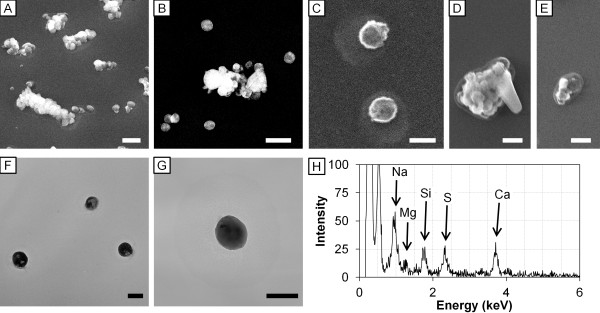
FE-SEM images, TEM images and EDS spectrum of particles released from ultrasonic humidifier. FE-SEM images of particles (0.5 − 2.5 μm) released from the humidifier with (A) tap water and (B) high-silica water under humidity 75%; particles (<0.5 μm) from the humidifier with tap water under (C) humidity 75% and (D, E) 99% are shown. TEM image of particles (<0.5 μm) from the humidifier with (F) tap water and (G) high-silica water under humidity 75%. Scale bars represent (A, B) 1 μm, (C, F, G) 200 nm, and (D, E) 500 nm. (H) EDS spectrum of the particle (C) is shown. The peaks of Na, Mg, Si, S, and Ca were detected at 0.97, 1.26, 1.78, 2.33, and 3.71 keV, respectively.
